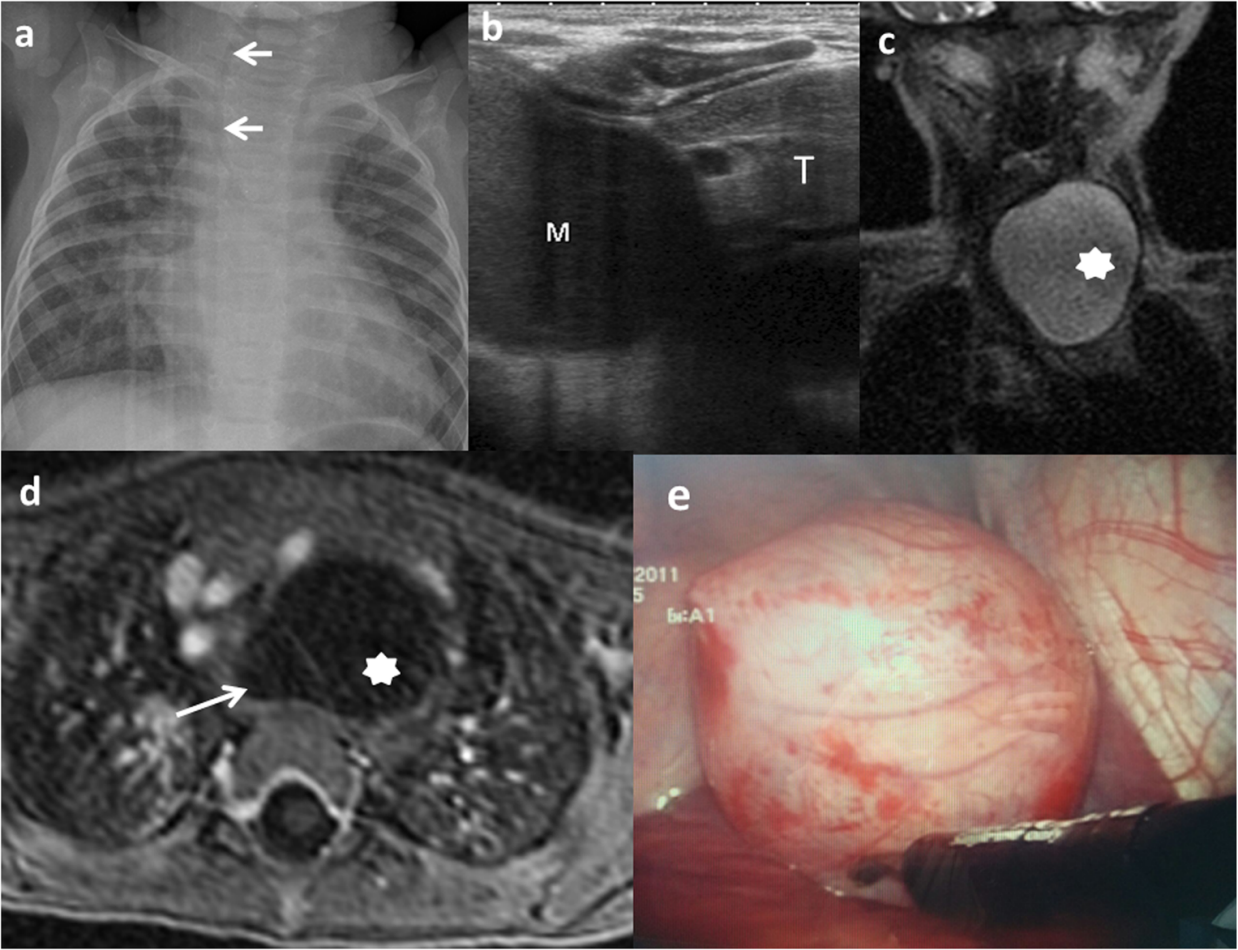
A 10-month-old boy with a congenital cardiopathy presents respiratory distress. a Chest X-ray: a left cervicothoracic mass is suspected displacing the trachea to the right (*arrows*). b Chest US: cystic mass (*M*) with slightly echogenic content inside is seen next to the thymus (*T*). Its origin is unclear. c Coronal view SSFSE T2 MRI: a well-delineated and hyperintense lesion (*star*) is seen. d Transversal view of the lesion in a gadolinium-enhanced VIBE MRI confirms the cystic nature of the mass (*star*) next to the anterior oesophageal wall (*arrow*). e Thoracoscopic findings: a 6 × 4-cm lesion with close contact with the trachea originated from the muscular wall of the oesophagus
